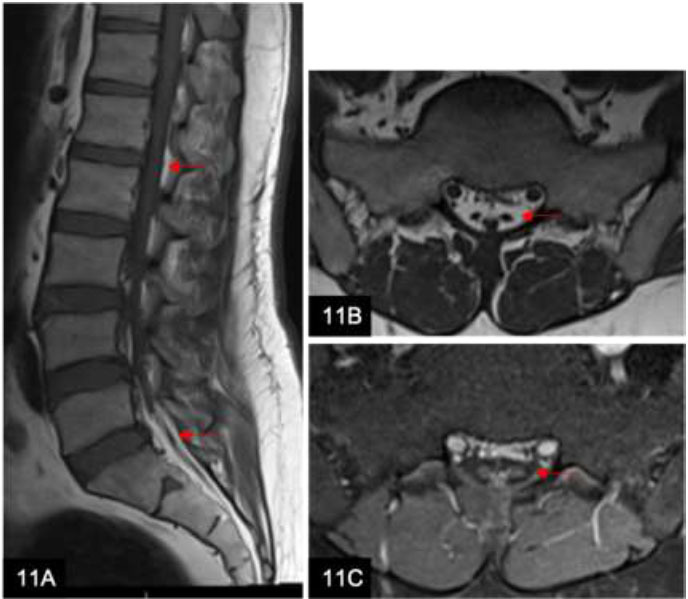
A 34-year-old man with a history of Guillain-Barré Syndrome presents with worsening lower limb weakness. Sagittal T1w (A), axial T1 (B), and axial T1w post-contrast (C) MRI lumbar spine scans show epidural lipomatosis with high signal intensities (A and B arrows) surrounding the cauda equina and lumbosacral nerve roots, mimicking leptomeningeal enhancement.
